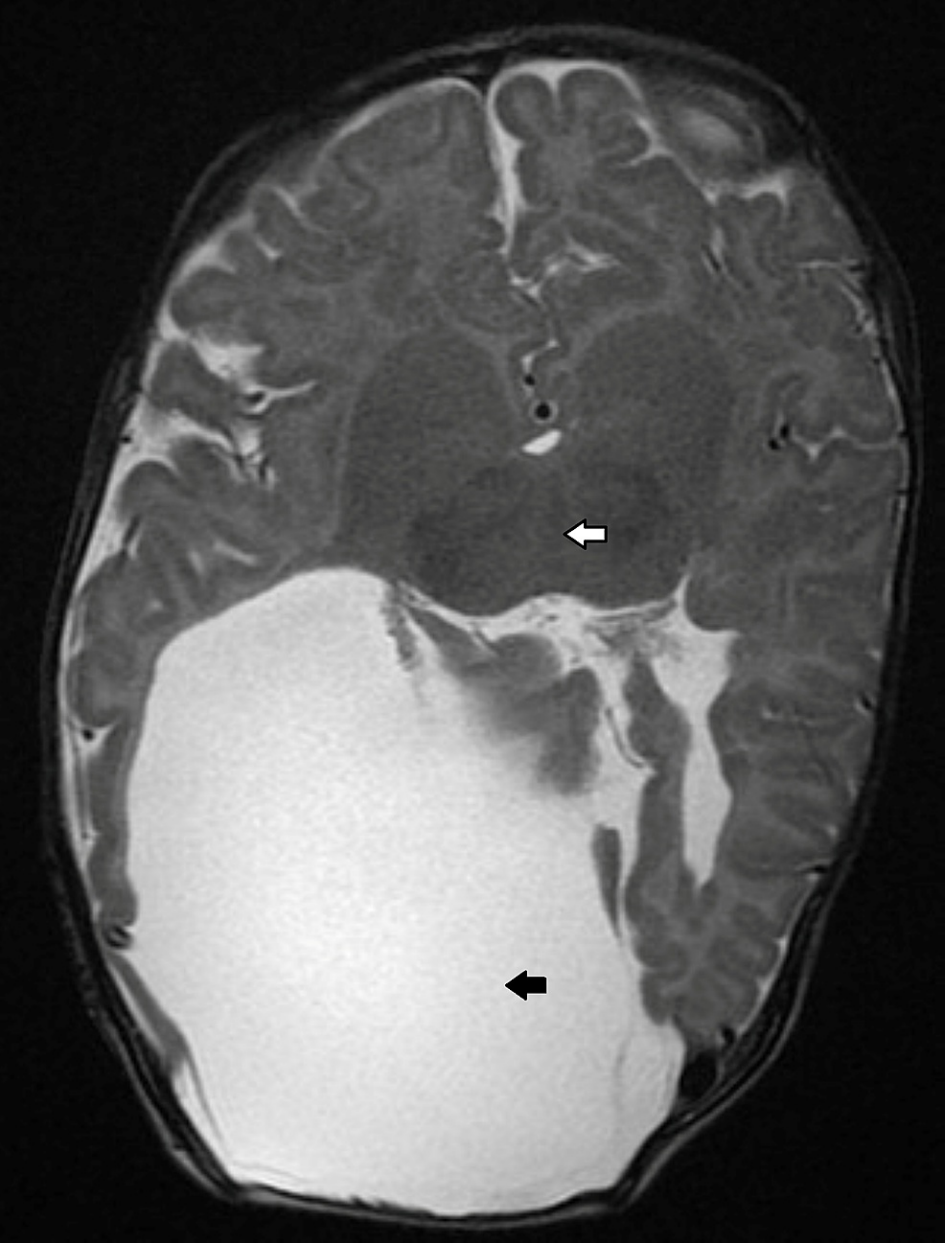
Axial section through cranial T2 MRI showing holoprosencephaly (white arrow) and schizencephaly (black arrow).
